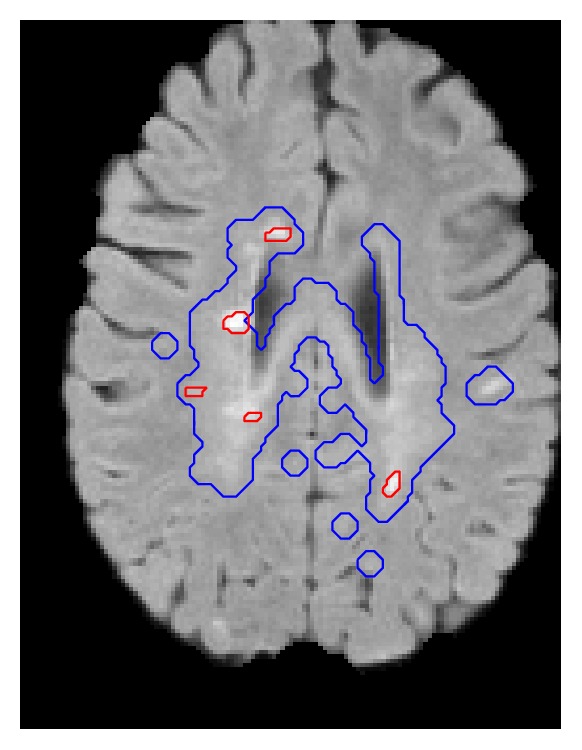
Candidate lesion regions mask (blue) and the lesions (red) on a FLAIR image.
